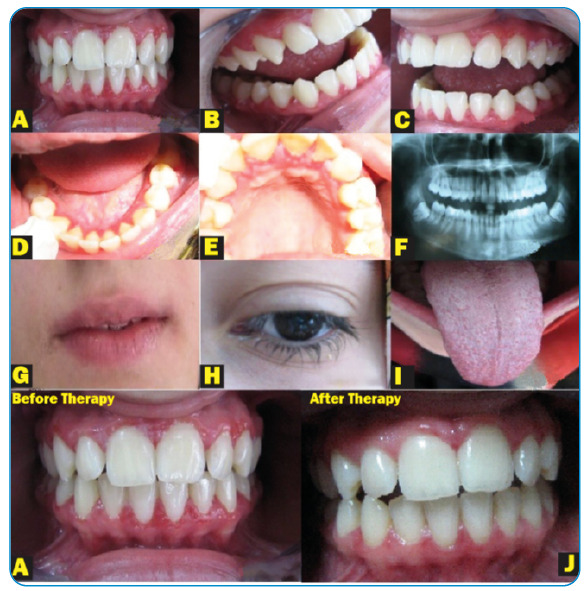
(A) Initial view. Note reddish/whitish lesions and peeling of the maxillary and mandibular attached gingiva. (Before therapy); (B) initial clinical intraoral view of the associated right region; (C) initial facial view of the associated left region; (D) clinical lingual view of the anterior mandible. Note diffuse erythema present on the gingiva; (E) clinical palatal view of the anterior maxillary. Note diffuse erythema and ulcer present on the gingiva; (F) panoramic radiograph view; (G) initial view. Note cheilitis present on the lips; (H) initial clinical aspect of the patient’s eye (conjunctivitis); (I) clinical aspect of the patient’s tongue (strawberry tongue); (J) view of the case 14 days after all therapy. The regular appearance was established (after therapy).
